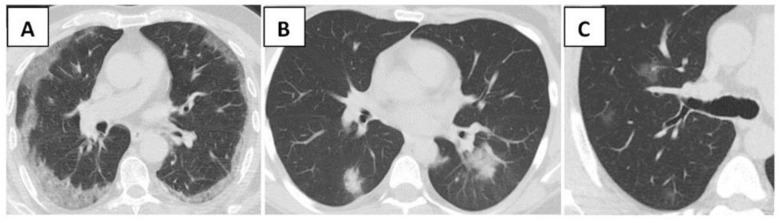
Chest CT findings of COVID-19. Transaxial images show (A) Bilateral, peripheral GGO; (B) Bilateral consolidation; (C) Multifocal rounded GGO. CT: computed tomography, GGO: ground-glass opacity.
